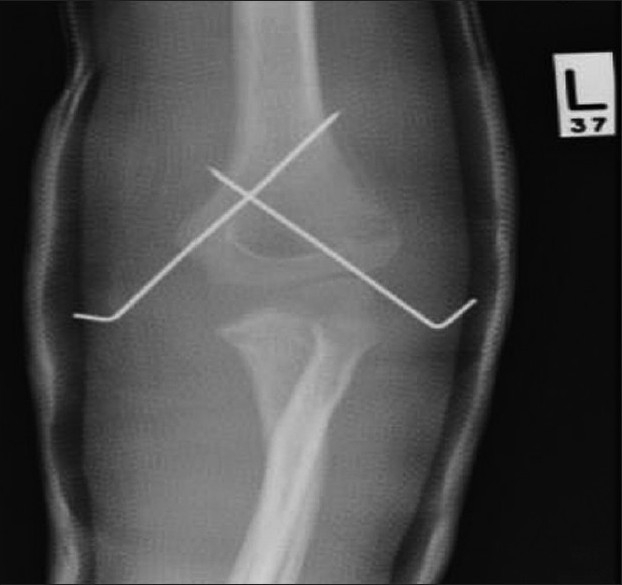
Anteroposterior radiograph demonstrating satisfactory fracture stabilisation
